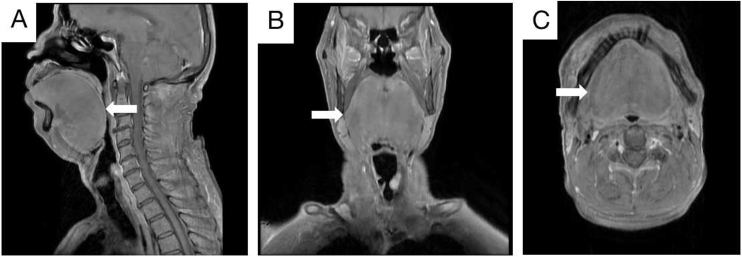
Enhanced MRI scan of nasopharynx and neck. The soft tissue of the tongue was obviously swollen, and the pharynx was compressed and narrow. No obvious signs of space occupation were observed. Sagittal position (A; White arrow). Coronal position (B; White arrow). Axial position (C; White arrow).
